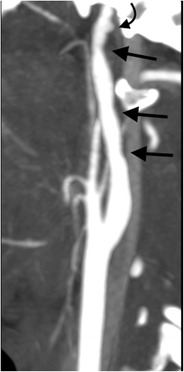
Blunt traumatic vascular dissection injury: right sagittal oblique reformatted image of CT angiography of a young female pedestrian who sustained Hangman’s fracture as a result of a “hit-and-run” motor vehicle accident demonstrates vascular dissection abnormality of the distal cervical segment of the right internal carotid artery below the skull base (*straight arrows*) with associated pseudoaneurysm formation (*curved arrow*)
